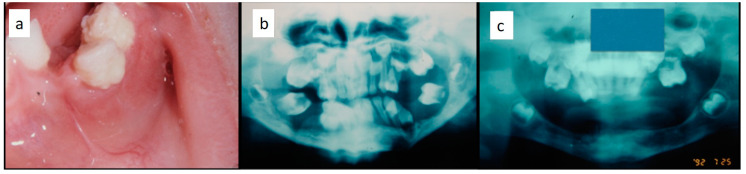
(a–c): Diffuse periodontal lesions in the mandible in a 5 year-old female patient causing teeth mobility with pathological fracture and dislocation on the left side (a,b); panoramic radiograph after medical and functional treatment (c).
